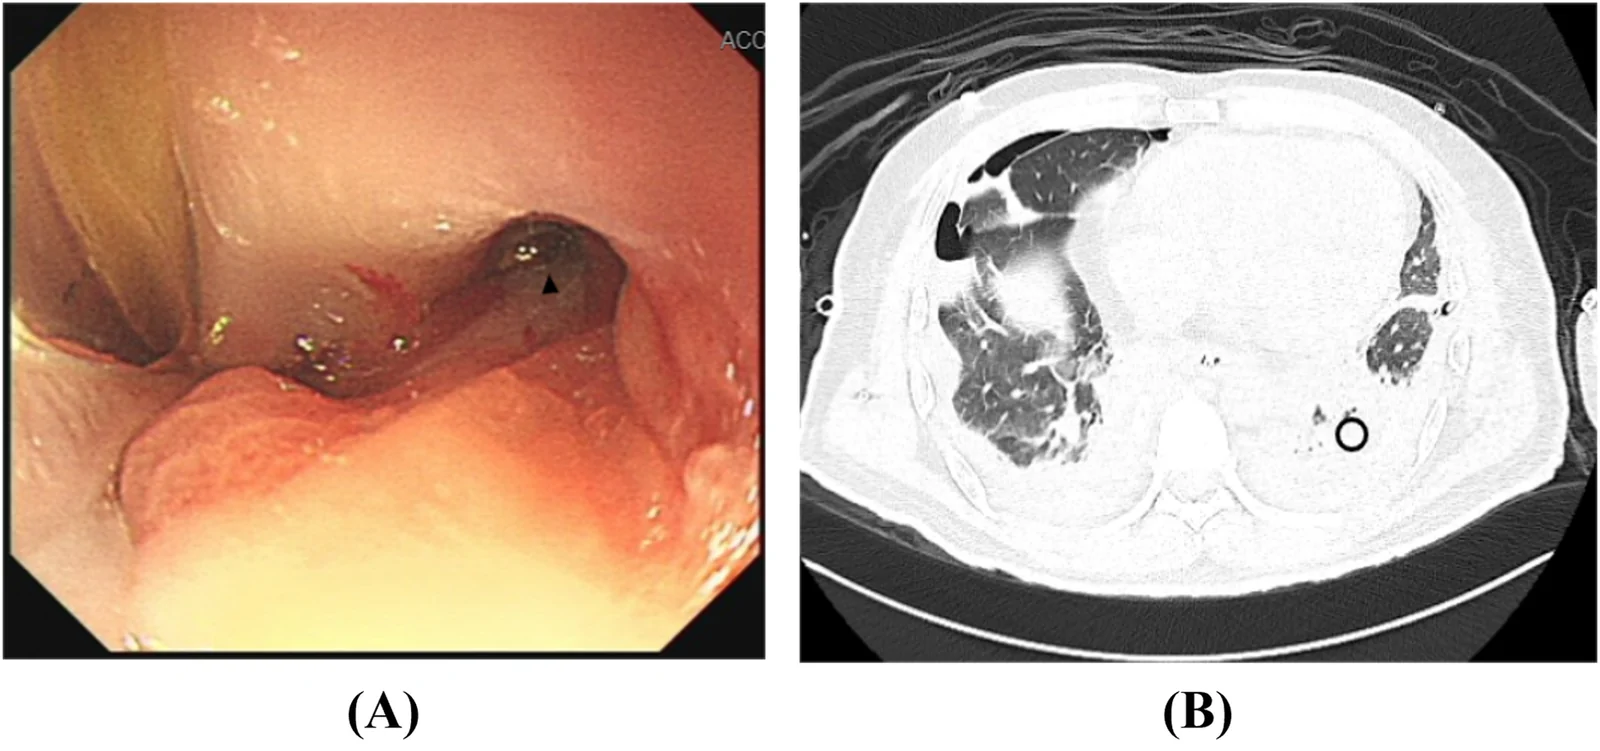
Identification of the esophageal perforation and persistent thoracic contamination. (A) Esophagogastroscopy demonstrating a distal esophageal perforation. (B) Follow-up chest CT showing persistent left pleural effusion and associated atelectasis (○).
